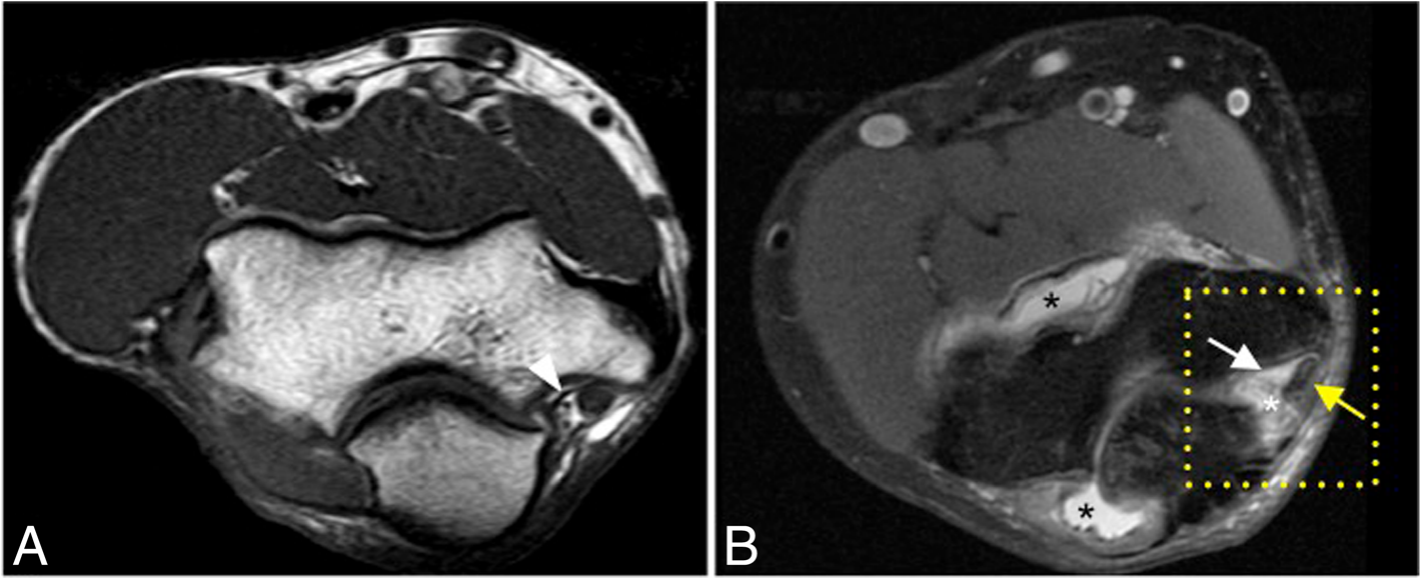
A 25-year-old man with pain and medial instability after a fall on an outstretched hand. Axial T1-weighted MRI (a) showing the intact right posterior bundle of the medial collateral ligament complex of a healthy volunteer (white arrowhead). Axial FS PD-weighted MRI (b) showing an acute tear of the left posterior bundle of the medial collateral ligament complex (white arrow), inflammatory changes in the soft tissue of cubital tunnel (white asterisk), displacement and flattening of the ulnar nerve (yellow arrow), and joint effusion (black asterisks)
